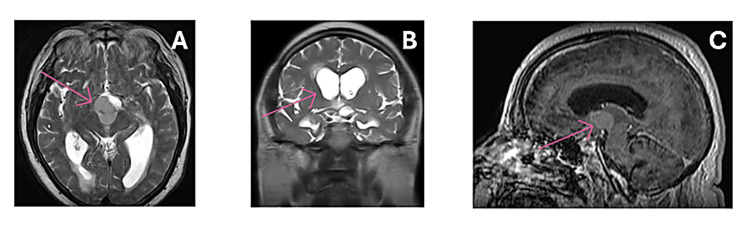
Preoperative MRI scans, prior to initial transventricular endoscopic cyst drainage. A: shows the acute hydrocephalus on an MRI (axial slice) performed on admission. The red arrow points to the suprasellar lesion. B: acute obstructive hydrocephalus (ventricular dilatation) shown on the coronal MRI slice. This was secondary to the large cystic craniopharyngioma lesion pictured in image C. C: shows the large suprasellar mass extending into the third ventricle on a sagittal MRI slice, causing the secondary acute obstructive hydrocephalus.
